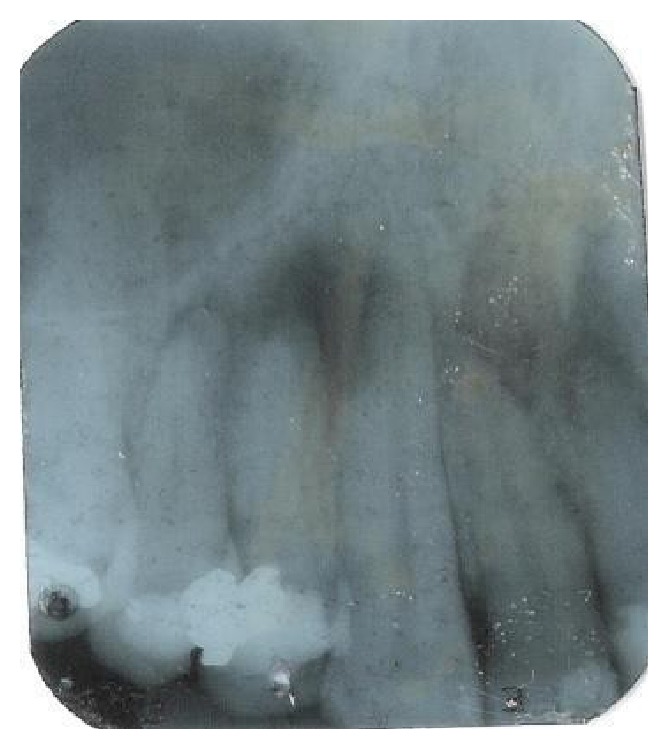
Periapical view of the right premolars. Extensive amalgam restorations on teeth 14 and 15 are observed; this location is close to the region of the apical periodontitis.
